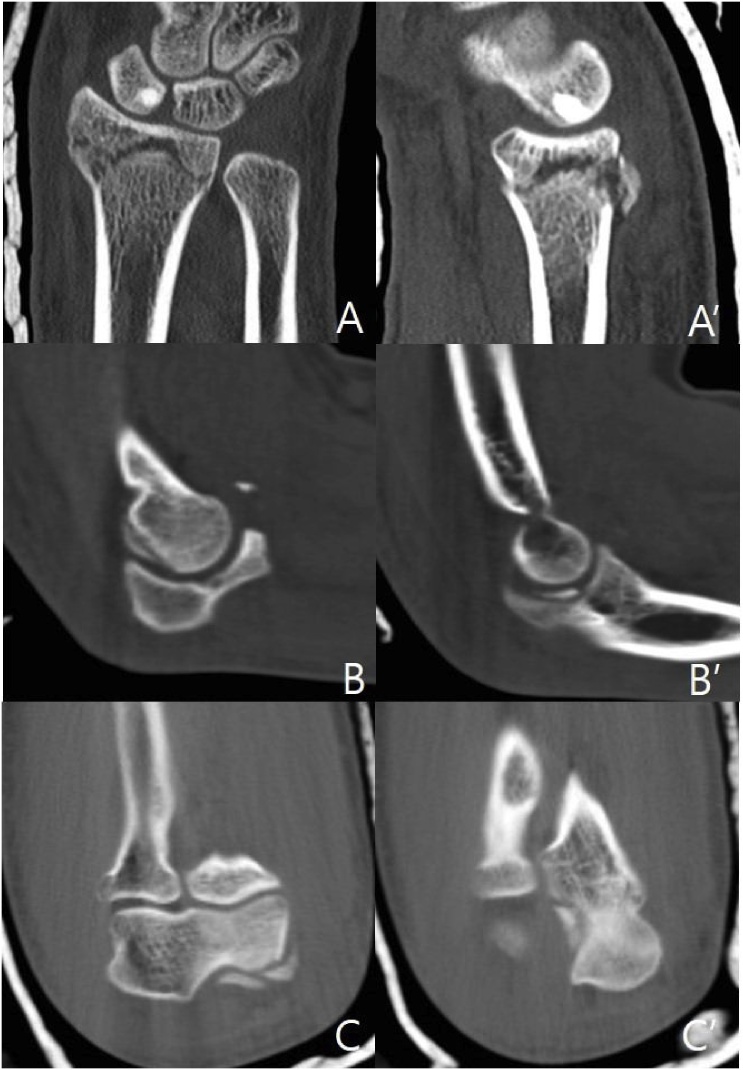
A and A’ CT scan of the left wrist demonstrate AO/OTA classification type A3 distal radius fracture. B and B’ Sagittal CT scan of the left elbow joint demonstrate coronoid tip fracture, trochlea osteochondral fracture, and intraarticular osseous body in elbow joint. C and C’ Axial CT scan of the left elbow joint demonstrate trochlea osteochondral fracture, and intraarticular osseous body in elbow joint.
